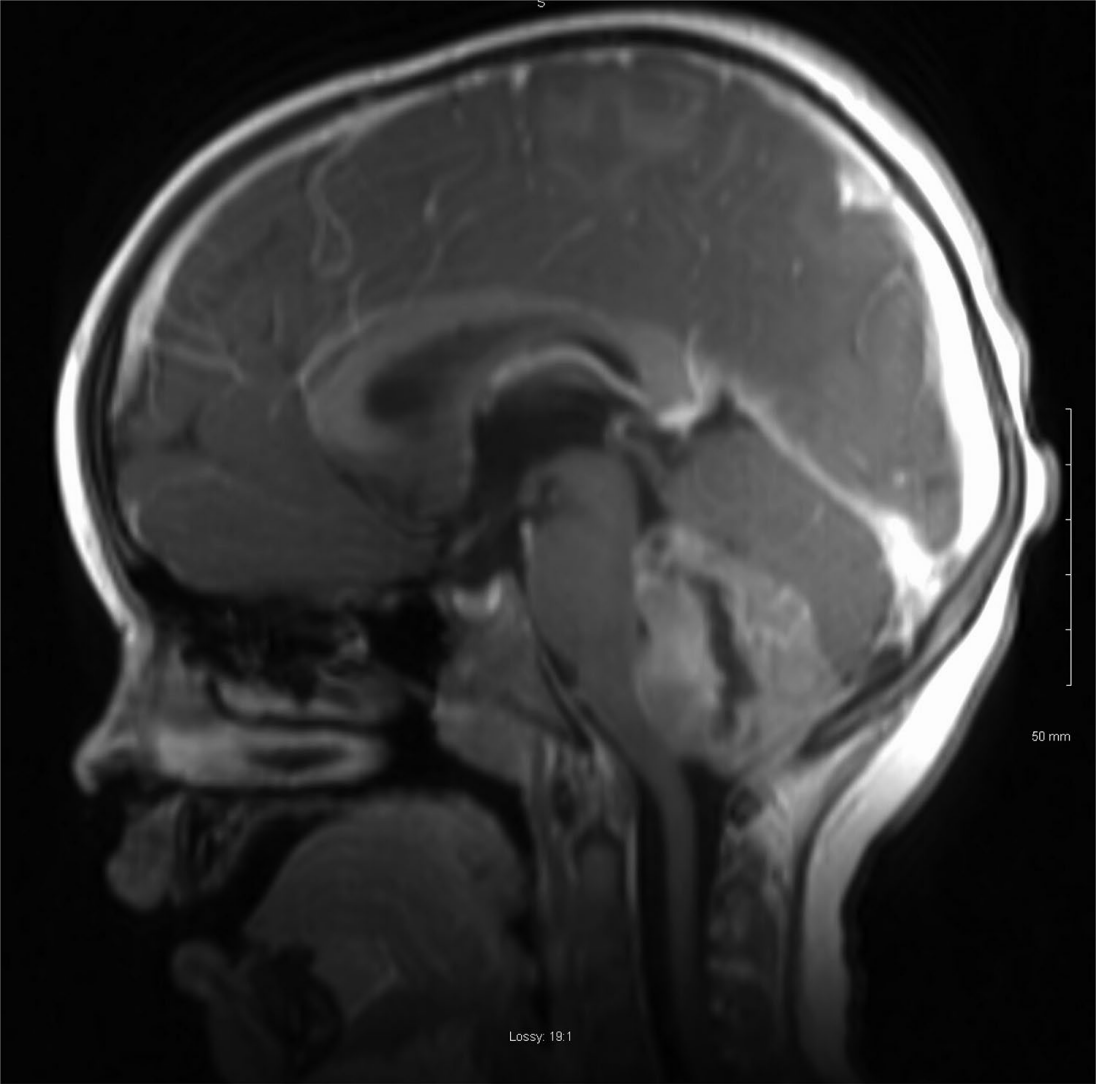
Preoperative MRI brain (sagittal view) of fourth ventricle tumour
